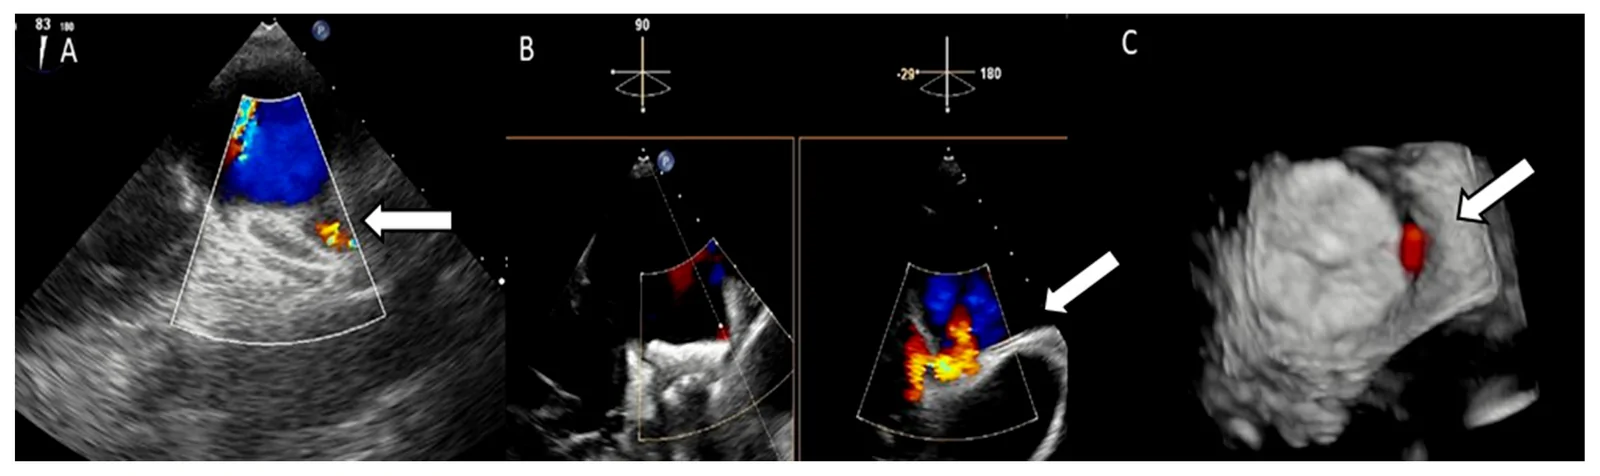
A colorDoppler interrogation of the LAA device (A). TEE demonstration of the device in two orthogonal planes showing a residual peri-device leakage (PDL) (B). A 3D color Doppler en face view of the device demarcating the crescent-shaped nature of the leakage (C).
